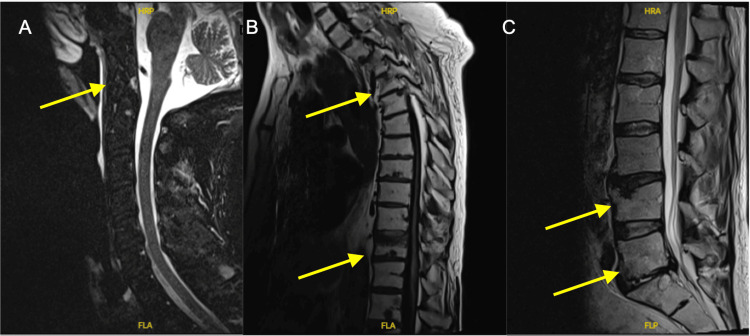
MRI spine images showing lytic lesions marked with yellow arrows. A: cervical spine lesions; B: thoracic spine lesions; C: lumbar spine lesions
